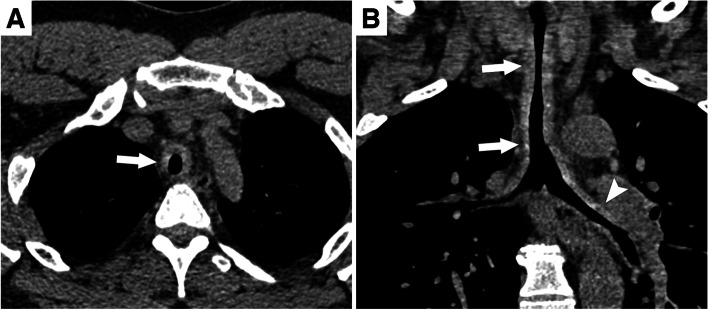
Relapsing polychondritis. A Unenhanced chest computed tomography shows tracheal wall thickening sparing the posterior membrane and responsible for lumen stenosis (arrow). B On coronal reformation, the tracheal wall thickening is extensive (arrows) and extends to the left main bronchus (arrowhead)
